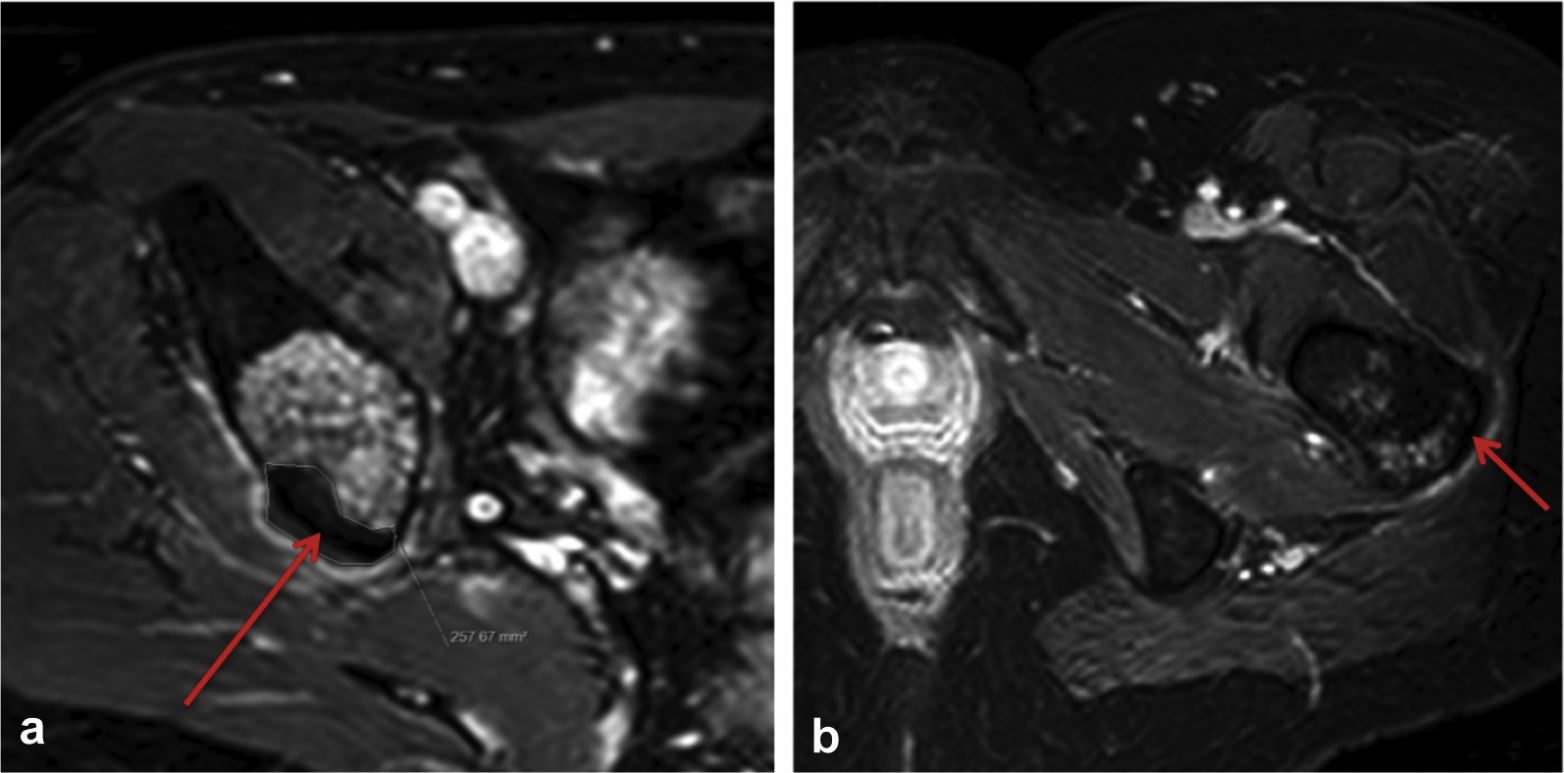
Example of a gadolinium-enhanced T1-weighted (Gd-T1W) image at 30 days after treatment. A clear focal region of nonenhancement (red arrows) was seen on either side of the bony cortex for intraosseous tumors (a) in a 36-year-old male with metastatic lung cancer, and (b) in a 45-year-old female with metastatic breast cancer. In both cases, a thin rim of enhancing tissue is also seen at the proximal border of the region of nonenhancement.
